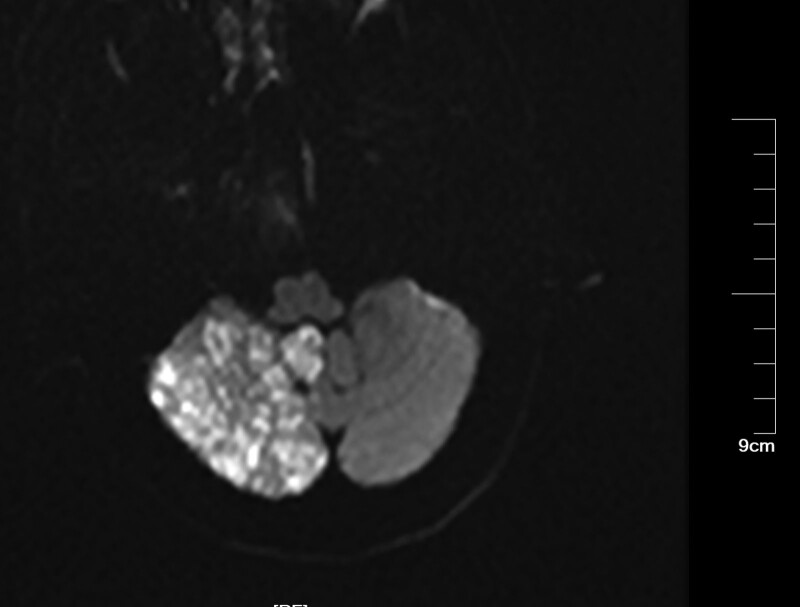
Follow-up DWI 2 days later demonstrated a significant high-intensity lesion in the right cerebellum. DWI = diffusion-weighted imaging.
